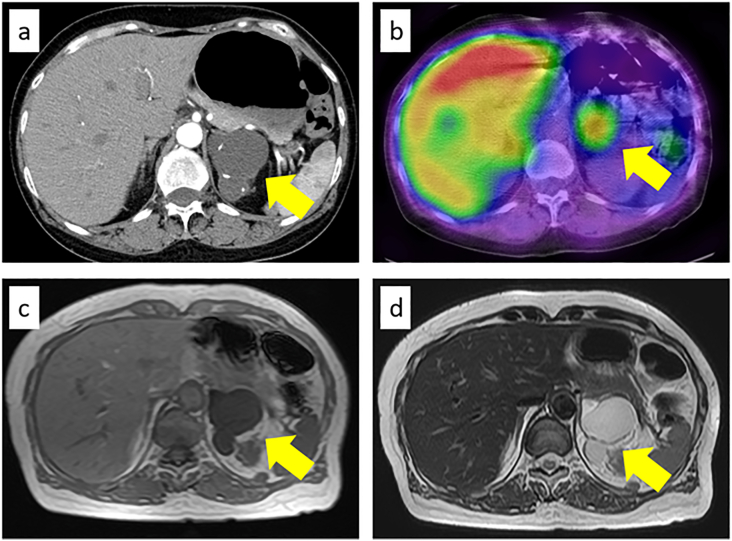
a Contrast-enhanced CT revealed a lobulated cystic lesion with calcification in the left adrenal gland. b 123I-MIBG scintigraphy showed intense uptake corresponding to the left adrenal cyst. c The lesion showed low signal intensity on T1-weighted MRI. d T2-weighted MRI demonstrated a 53-mm multilocular cystic lesion with septations and high signal intensity.
